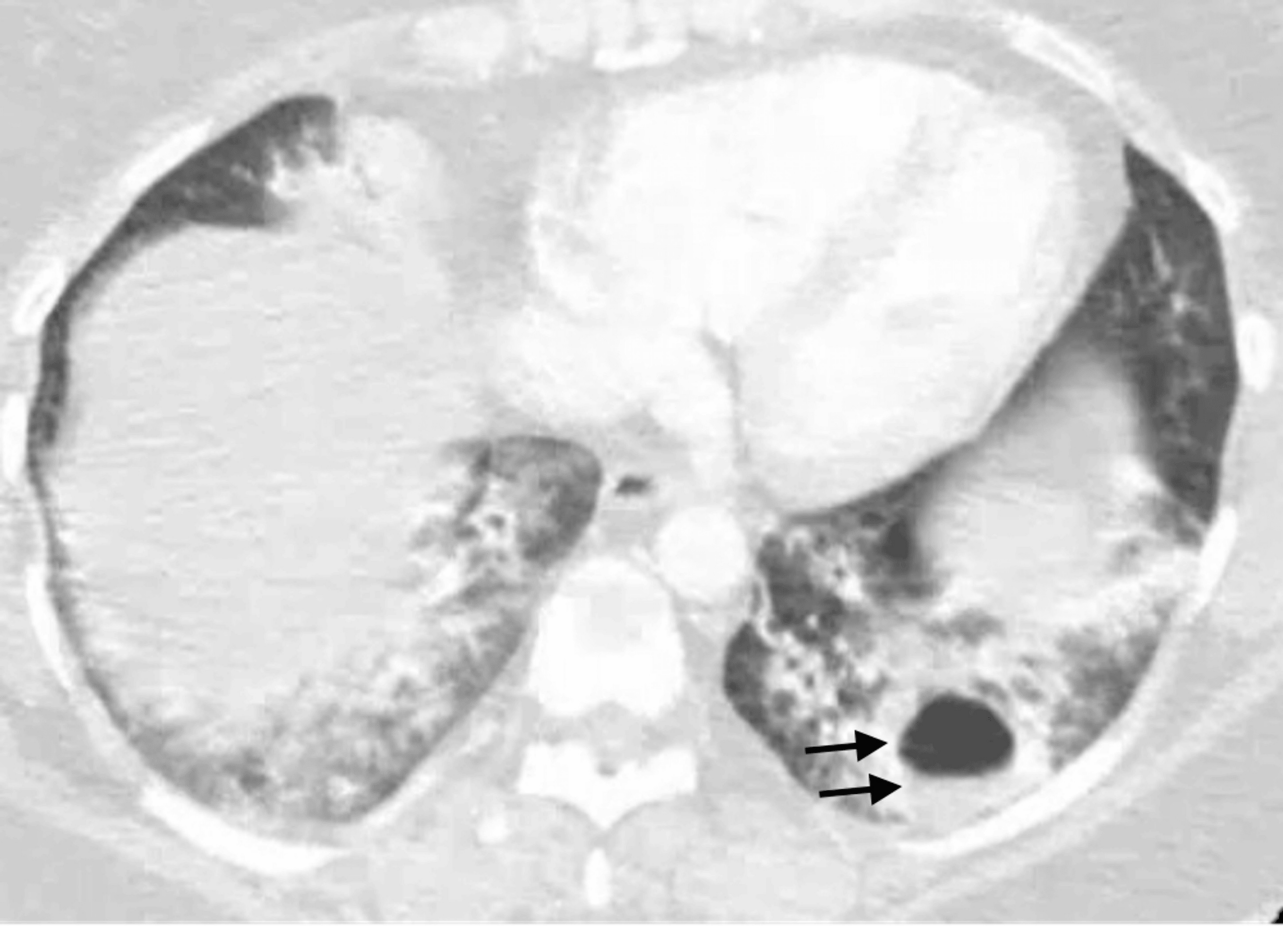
Chest CT showing left lower lobe cavitary lesion with air-fluid level and bilateral ground glass opacities
